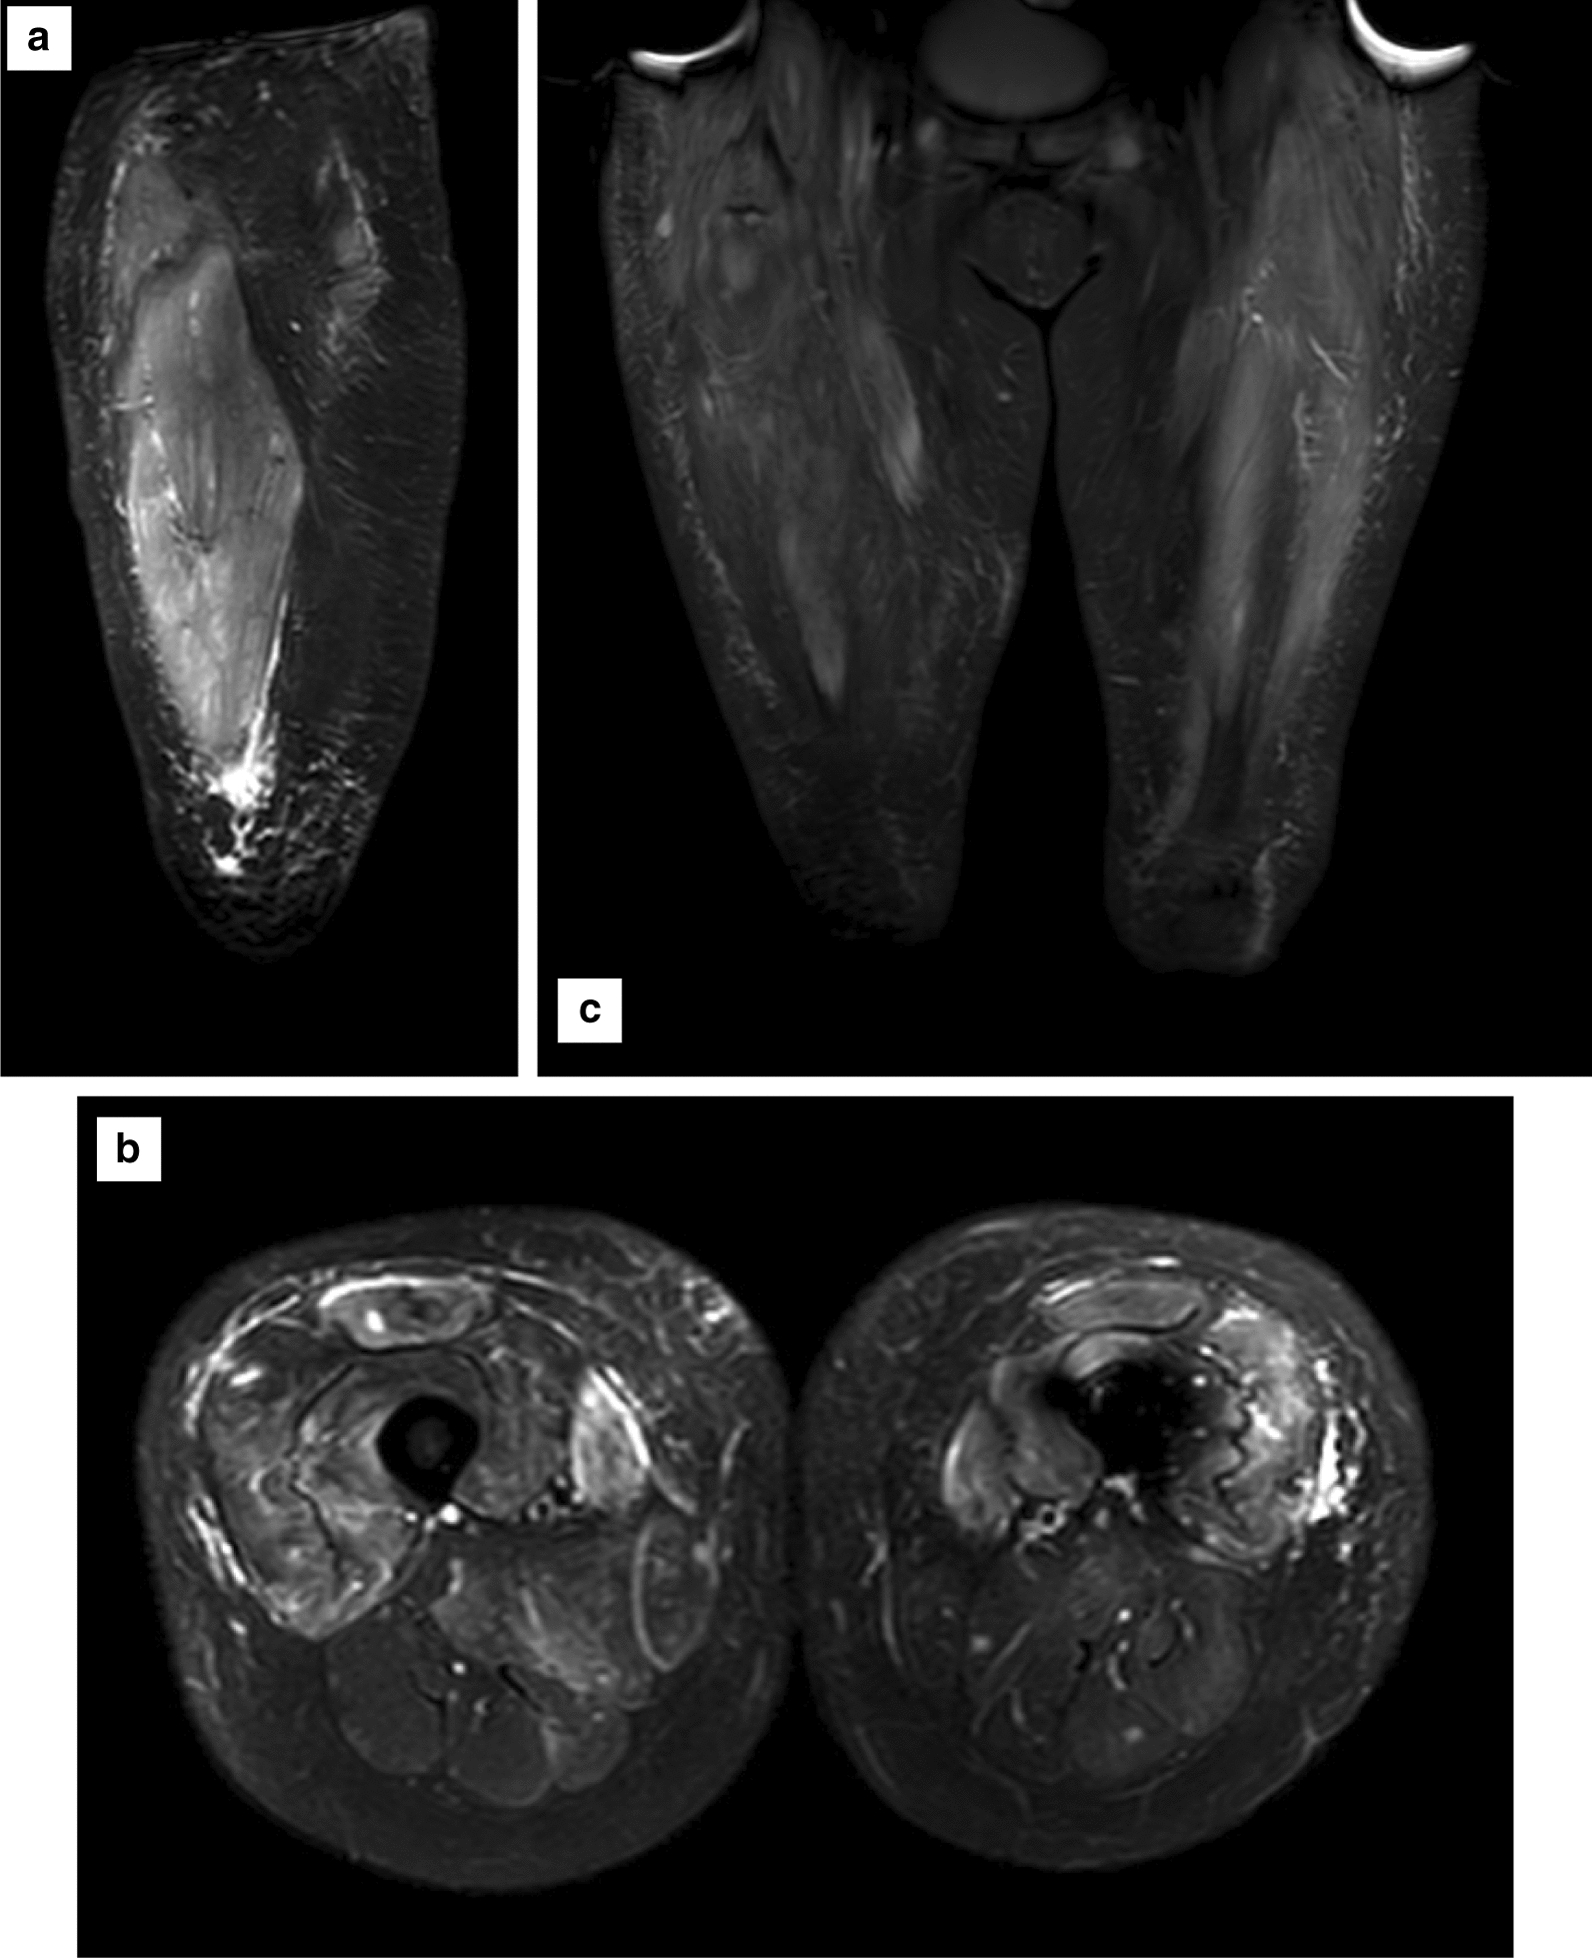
MRI signal change of the musculature of the lower limbs on (a) left sagittal (b) axial (c) coronal inversion recovery sequence in keeping with myositis
